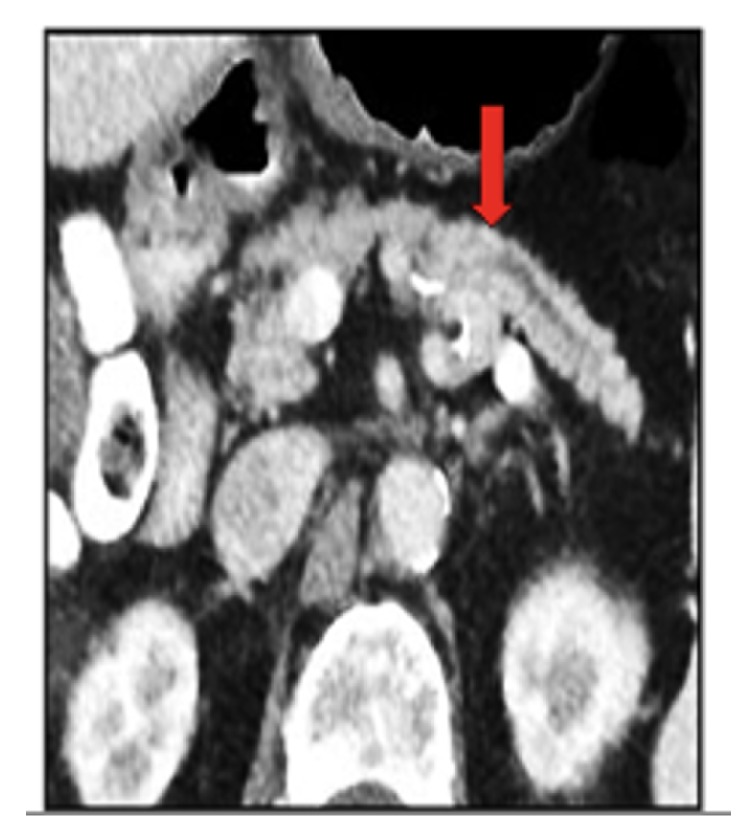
Axial abdominal CT-scan showing dilatation of the pancreas and duct cut-off sign (red arrow).
